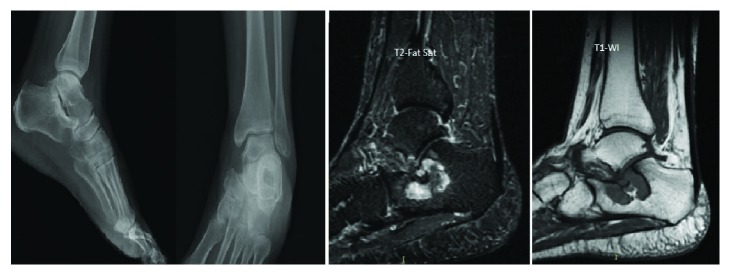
Postoperative plain radiographs and MRI (sagittal T1-WI and T2 fat sat sequences) reveal postsurgical changes in the calcaneus with decrease in the size of the mass which is partially replaced with marrow fat.
